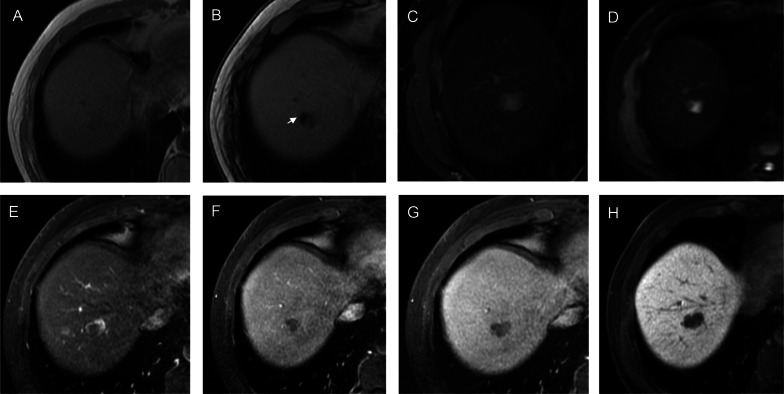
Gadobenate dimeglumine–enhanced axial MRI scans in a 57-year-old man with hepatitis B virus (A-H). A 1.7-cm steatotic mass is detected in segments VII. The mass shows T1 hypointensity (A), signal intensity reduced on out-of-phase TIWI (arrow in B), moderate T2 hyperintensity (C), marked hyperintensity on DWI (b = 1000 s/mm^2^) (D), rim enhancement on arterial phase image (E), “wash-out” appearance on portal venous phase (F) and equilibrium phase (G), marked hepatobiliary phase hypointensity (H). The recurrence-free survival for this patient was 150 days
